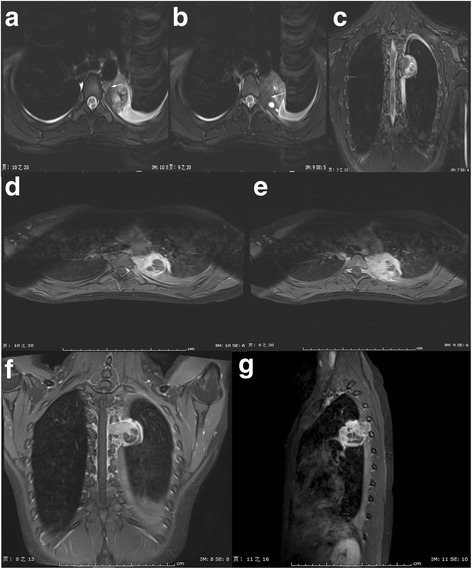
a–c Axial and coronal T2-weighted images showed a heterogeneous mass, whose main component was solid, mixed with small portion of cysts with different sizes. Moreover, inside the solid portion of the tumor, tiny septae of hypointensity (*white arrow*) were detected. d–g Gd-enhanced T1-weighted images showed intensely heterogeneous enhancement. The solid portion with septae inside and the walls and septae of the cysts displayed a significant enhancement
